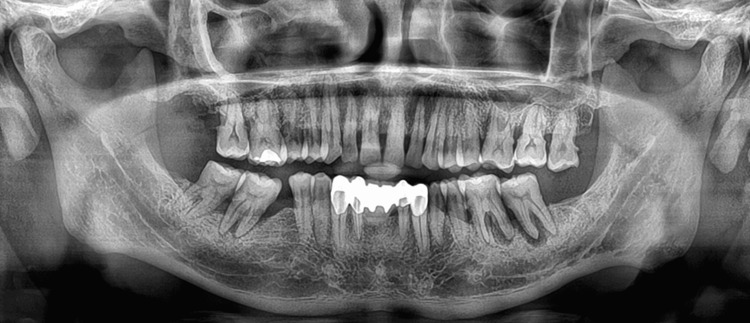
An orthopantomogram showing advanced periodontal disease with furcation involvement of right and left mandibular molars Image credit: Dr. Karthikeyan Ramalingam, Dr. Jayanth Kumar
